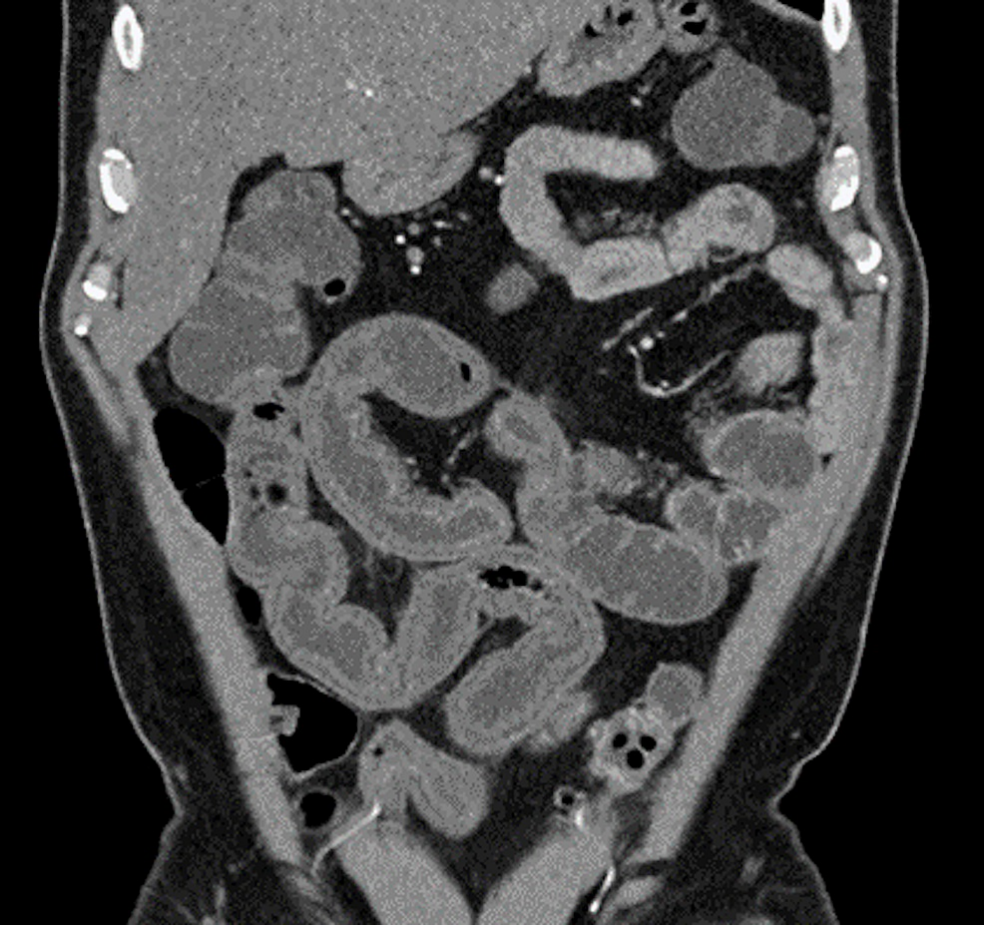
Computed Tomography Scan Computed tomography coronal view showed multiple loops of the small intestine with wall thickening, edema, and hyperenhancement.
